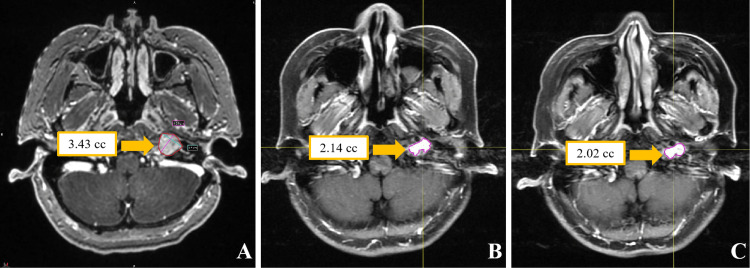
Contrast-enhanced MRI images of Case 2. (A) shows an axial contrast-enhanced T1-weighted image with contouring of the tumor volume before radiosurgery; (B) shows the same sequence with tumor volume contouring one year post-ZAP-X radiosurgery; (C) shows the axial contrast-enhanced T1-weighted imaging with tumor volume contouring three years post-ZAP-X radiosurgery.
